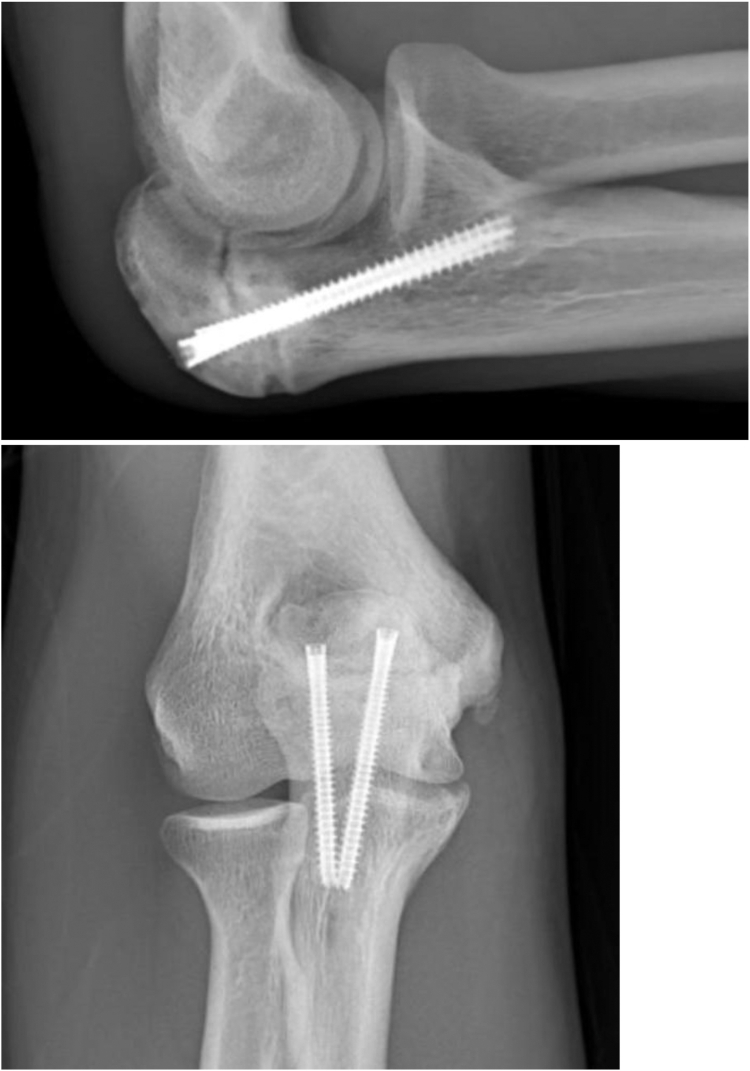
7-month postoperative plain films: continued well-maintained alignment of hardware with improved moderate callus formation and persistent sclerotic fracture edges.
